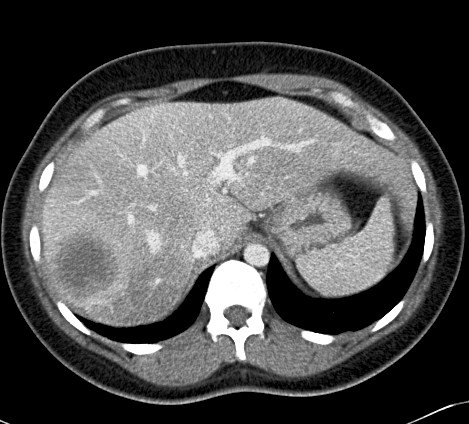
Abdominal CT image demonstrating a large mass lesion in the right hepatic lobe.
